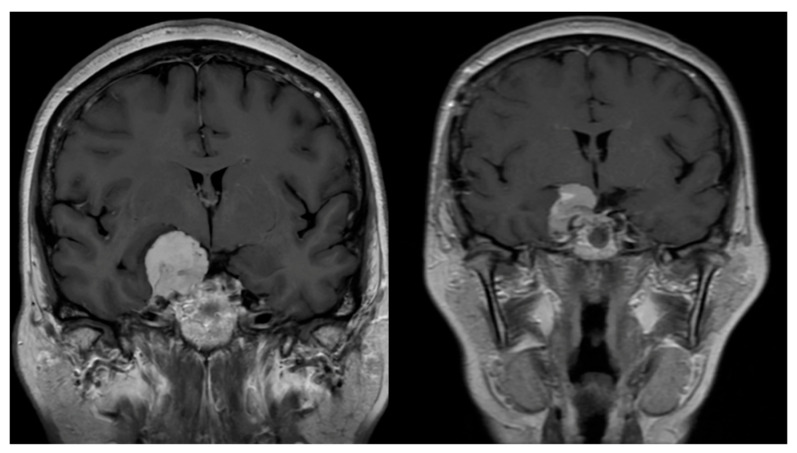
MRI study prior to surgical resection of the lesion (left), coronal MRI images using T1-weighted sequence with gadolinium contrast, and MRI study after surgical resection of the lesion (right), coronal MRI images using T1-weighted sequence with gadolinium contrast, where a decompression of the right temporal lobe can be observed.
